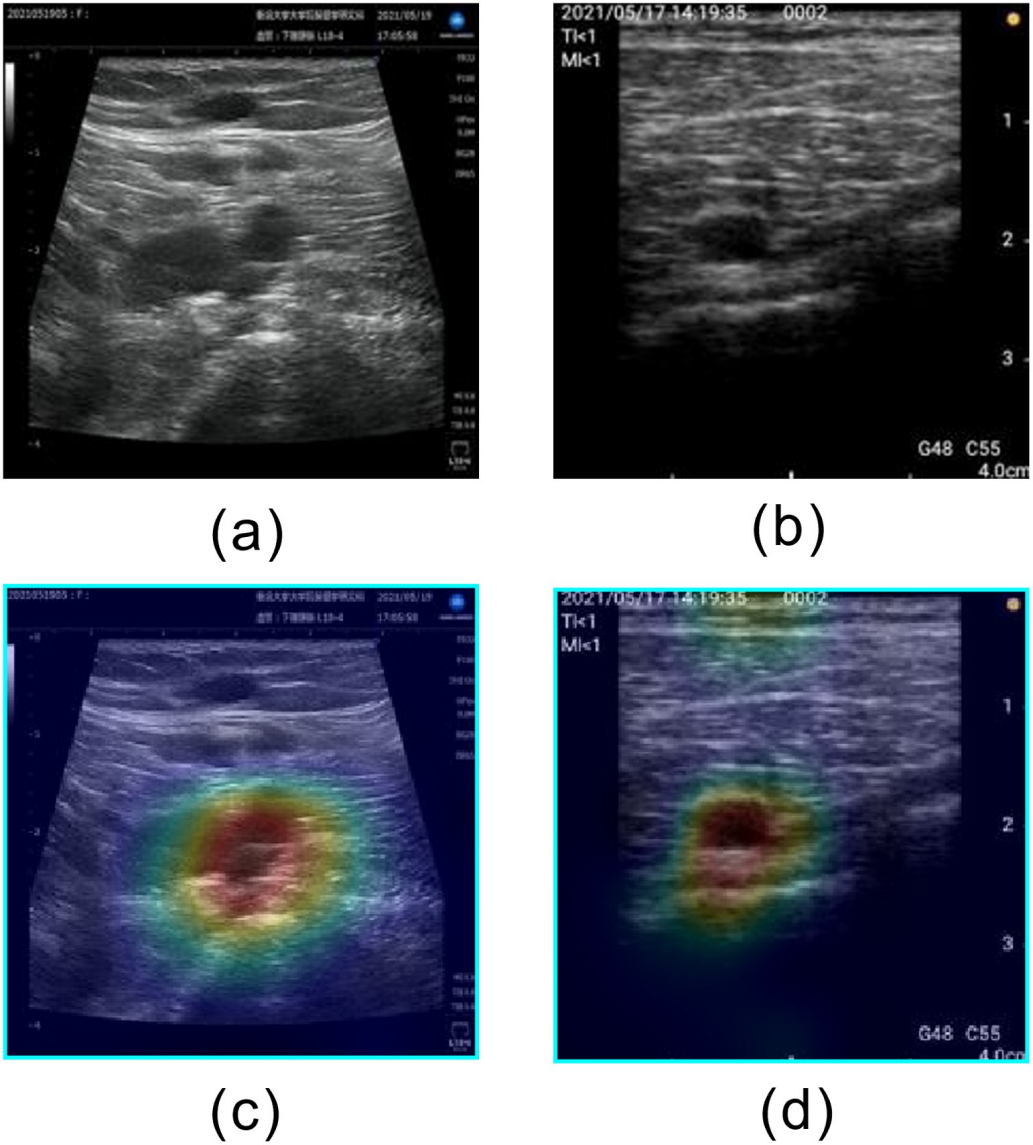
Examples of Moderately Satisfactory images misclassified as satisfactory images and the corresponding heatmap images. (a) Long axis acquired by stationary ultrasonography equipment, (b) Long axis acquired by Portable ultrasonography equipment, (c) Long axis acquired by stationary ultrasonography equipment, and (d) Long axis acquired by portable ultrasonography equipment.
